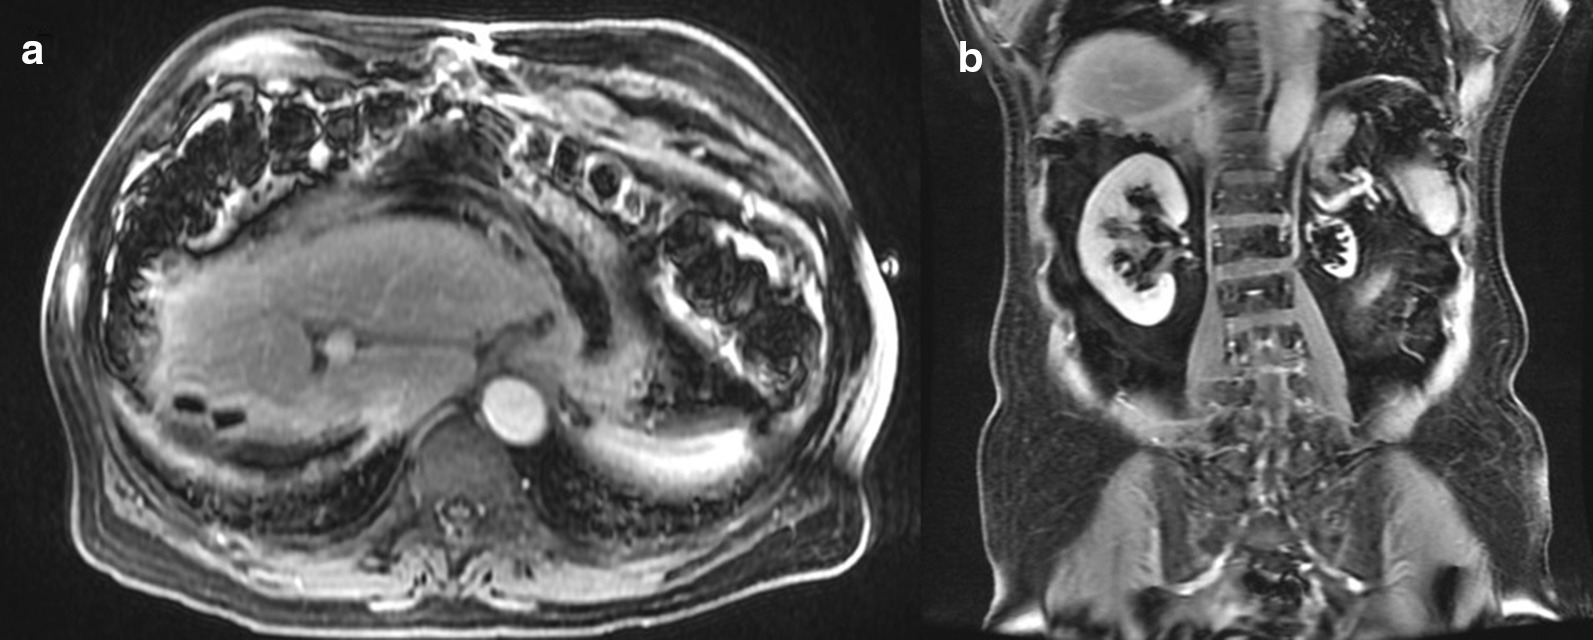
No recurrent disease on MRI 1-month post-partial right hepatectomy. a Axial and b coronal portal venous phase post-Eovist T1-weighted MRI images demonstrate blooming artifact from surgical clips in the right hepatic resection margin. Pathology did not demonstrate any viable tumor
